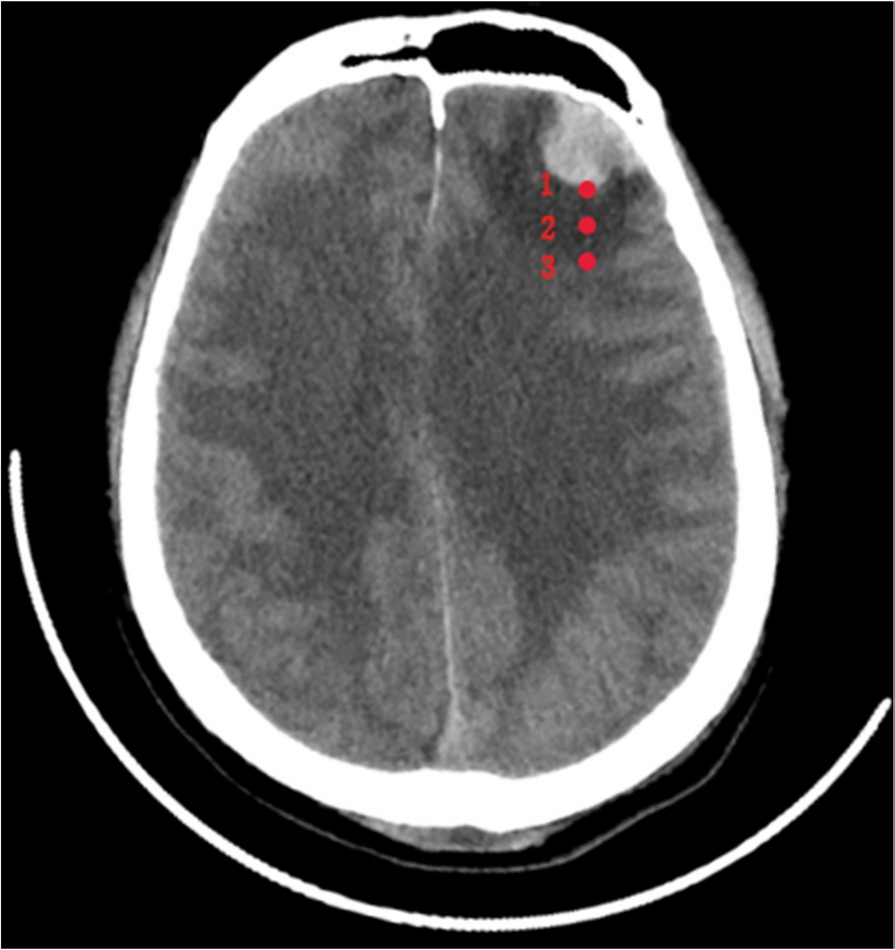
The maximum width of the edema zone around the contusion was selected, 3 points were equally spaced along the line and the corresponding CT values were recorded
